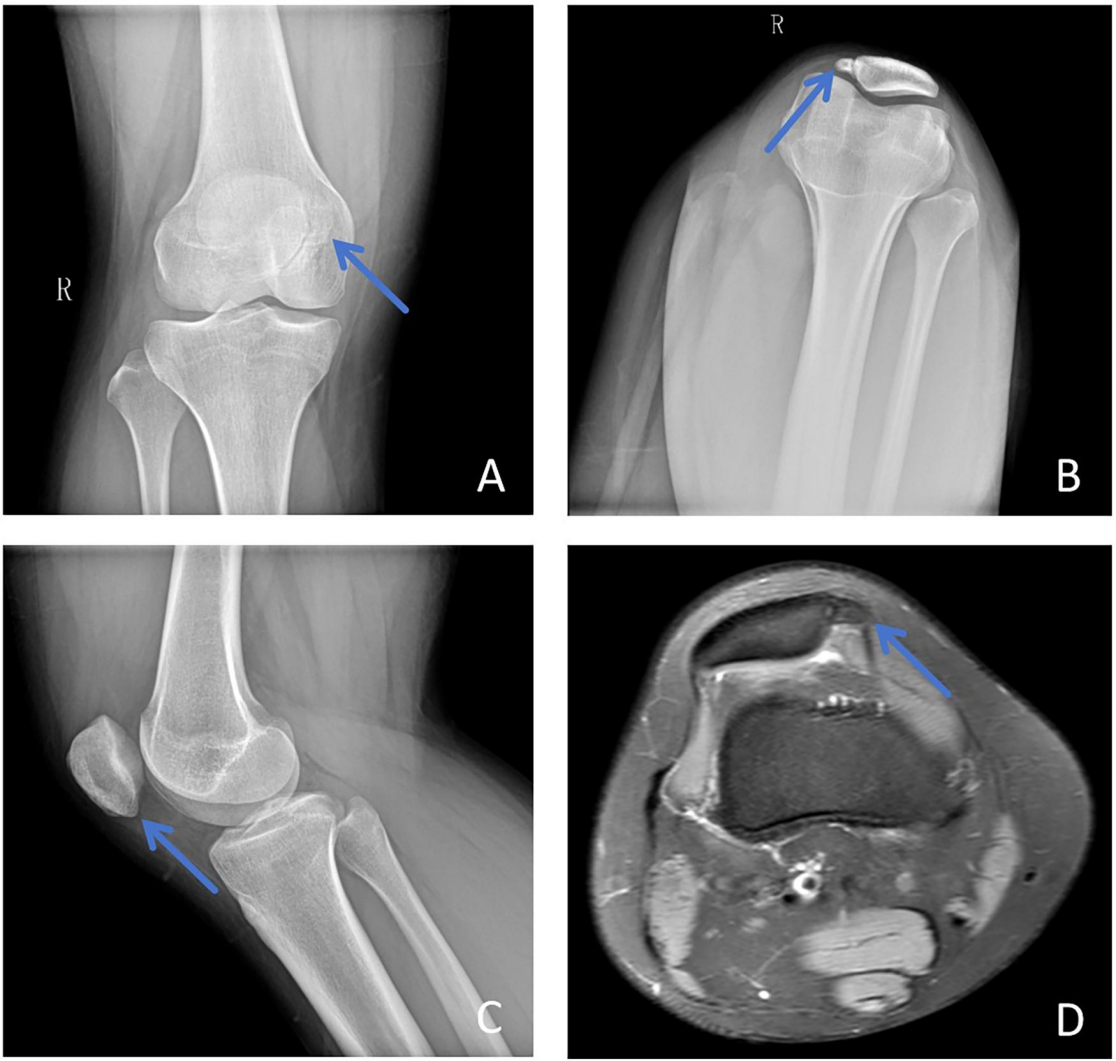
Preoperative imaging. (A) Anteroposterior view of the right patella. (B) Axial view of the right patella. (C) Lateral view of the right patella. (D) MRI of the right knee joint. On the anteroposterior and axial x-rays of the right patella (A,B), the cortical bone appears continuous and smooth, with a nodular high-density lesion adjacent to the patella. The lesion exhibits well-defined margins, consistent with a diagnosis of BP. The left patella shows no abnormalities on anteroposterior or lateral views (C) MRI of the right knee (D) demonstrates a high-riding patella with slight lateral displacement, accompanied by a small nodular lesion on the medial aspect exhibiting low signal intensity on both T1- and T2-weighted images.
